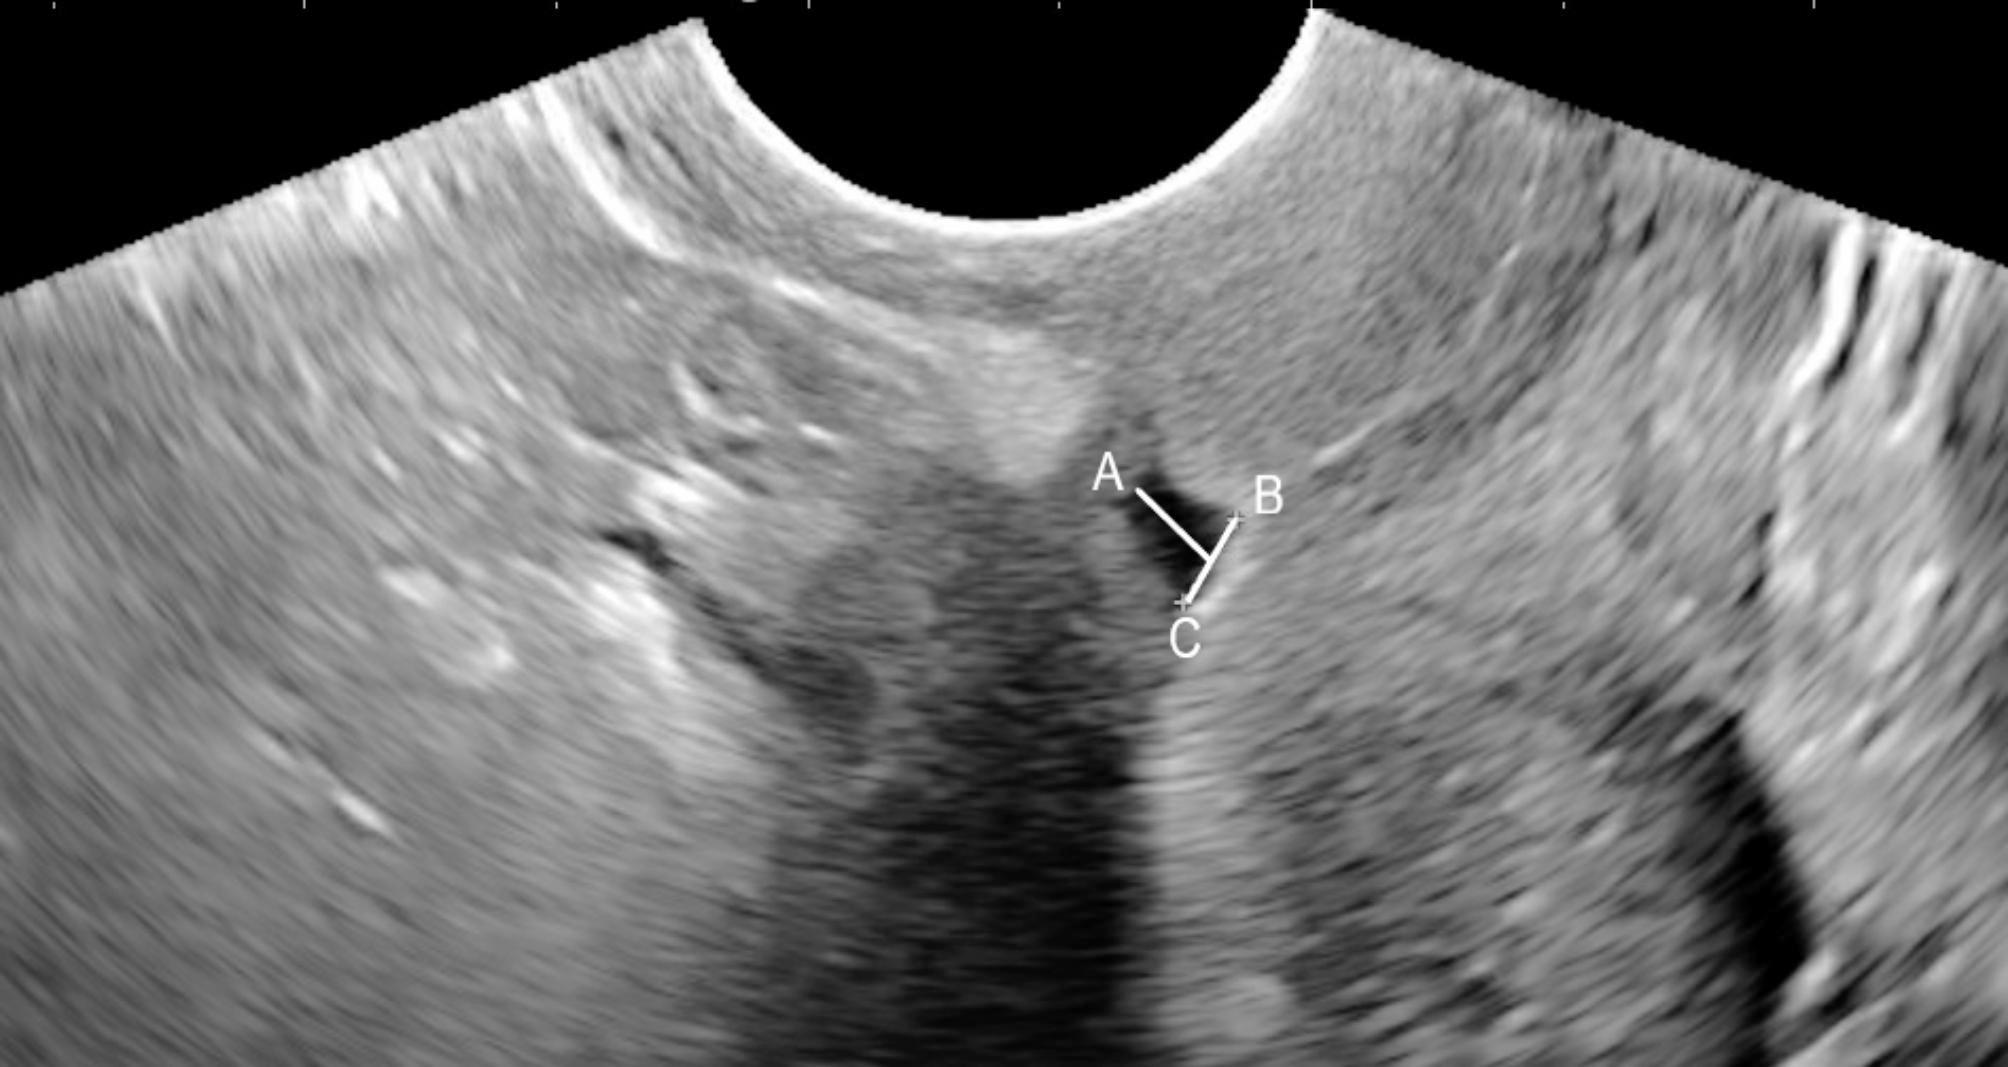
Transvaginal Ultrasonographic Appearance of a Cesarean Scar Defect in Sagittal View. The letter A represents the apex, while the BC line represents the base. The defect’s length is equal to the BC line’s length; the defect’s depth is defined as the distance between the apex and the middle of the BC line
